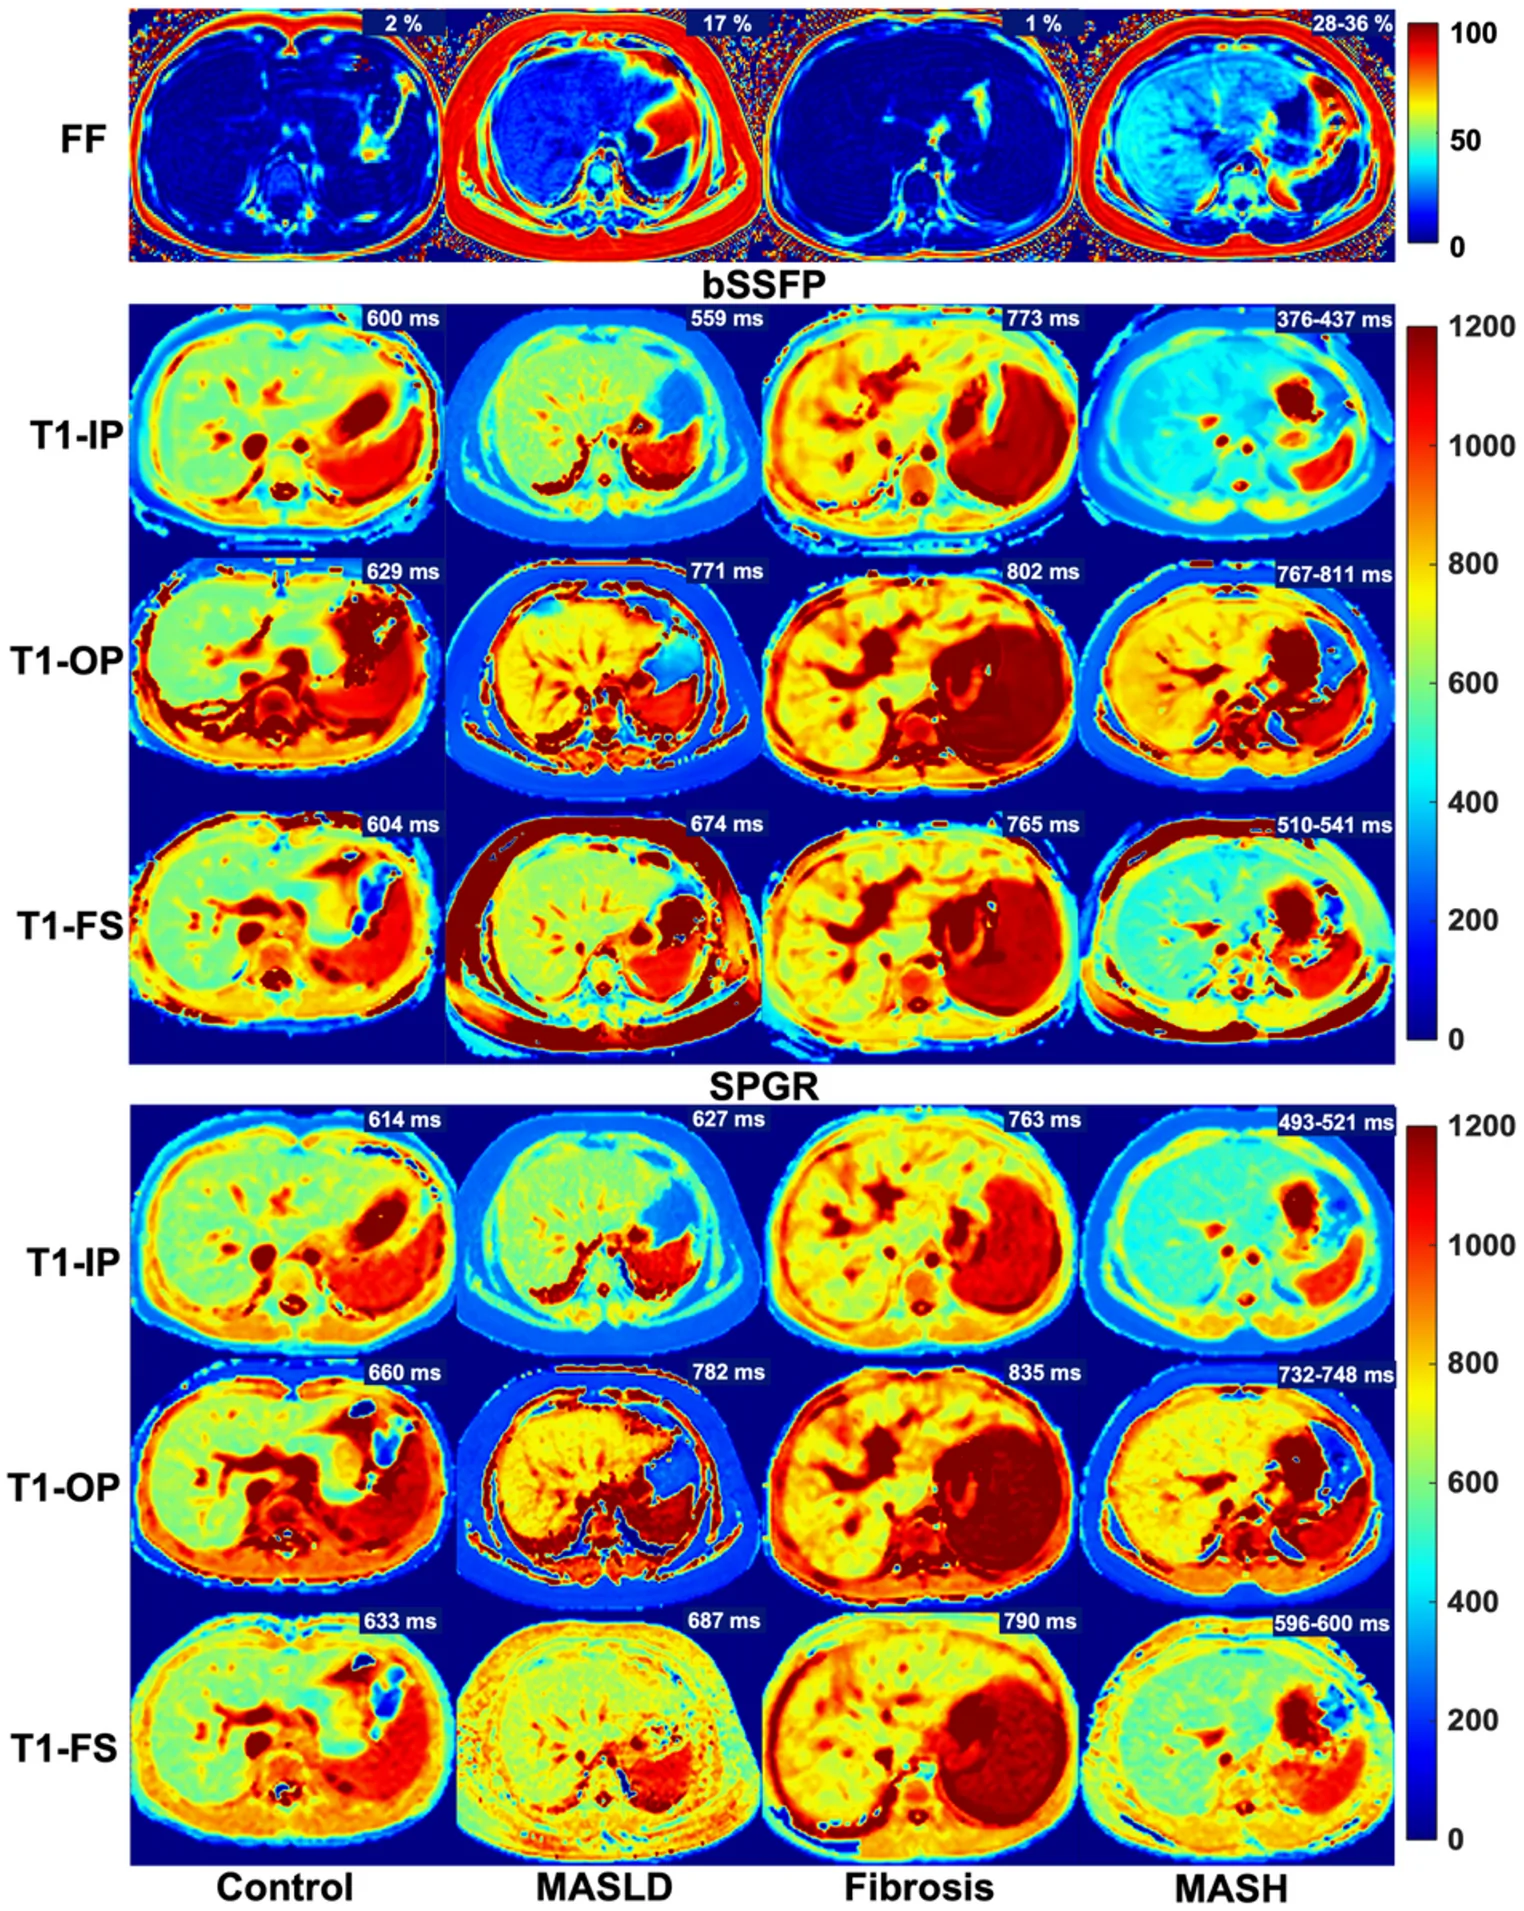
Representative maps from four participants (columns) showing proton density fat fraction (PDFF) from mDIXON Quant^®^ and T1 values obtained using MOLLI acquisitions with bSSFP (top) and SPGR (bottom) readouts. The top row displays PDFF maps from 3D six-echo mDIXON Quant^®^. The second, third, and fourth rows show T1 maps from MOLLI acquisitions using IP echo times, OP echo times, and FS, respectively. Numbers in top right show the mean of the three reader-averaged ROI values, or the range when the standard deviation of PDFF across the nine ROI measurements (three ROIs × three readers) exceeded 2%. Participants (left to right): control (15-year-old female); biopsy-confirmed MASLD/borderline MASH (10-year-old male); sclerosing cholangitis with advanced fibrosis (19-year-old male); and elevated liver enzymes with ultrasound shear wave speed of 1.9 m/s and clinical diagnosis of MASH (11-year-old male)
